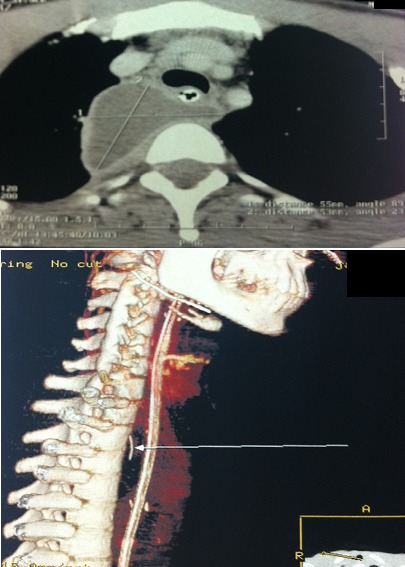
Cervicothoracic CT control objectifying migration of foreign body in the level of mediastinum with the presence of a mediastinal collection (A,B)
